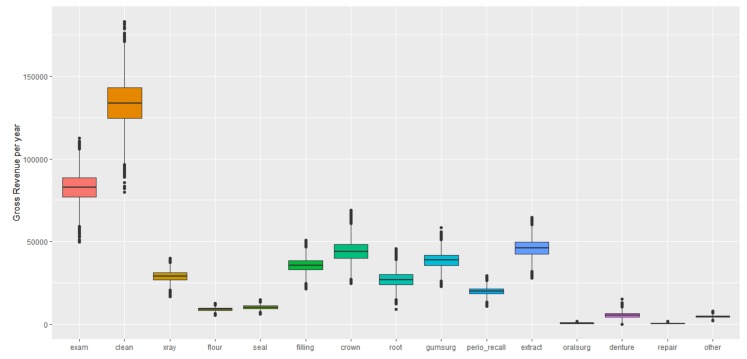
Gross revenue by procedure type, showing the minimum (lower whisker), maximum (upper whisker), median (center of the box), lower quartile (bottom of box), and upper quartile (top of box) values. Exam = diagnostic; clean: prophylaxis; X-ray = radiographic image; flour = fluoride; seal = sealant; root = root canal; gumsurg = periodontal scaling, root planning or gum; extract = extraction/ tooth pulled; repair = repair of bridges/dentures or relining.
